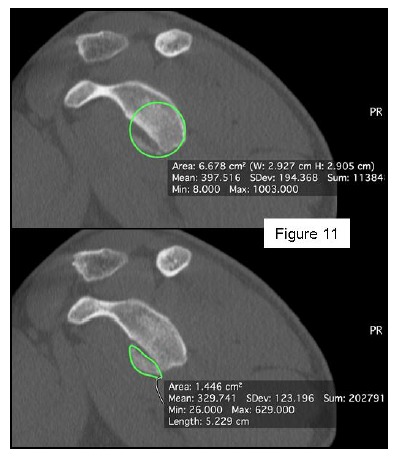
PICO method on 2D-CT en face view to quantify the glenoid bone defect area and percentage of bone loss. 87x101mm (150 x 150 DPI).
